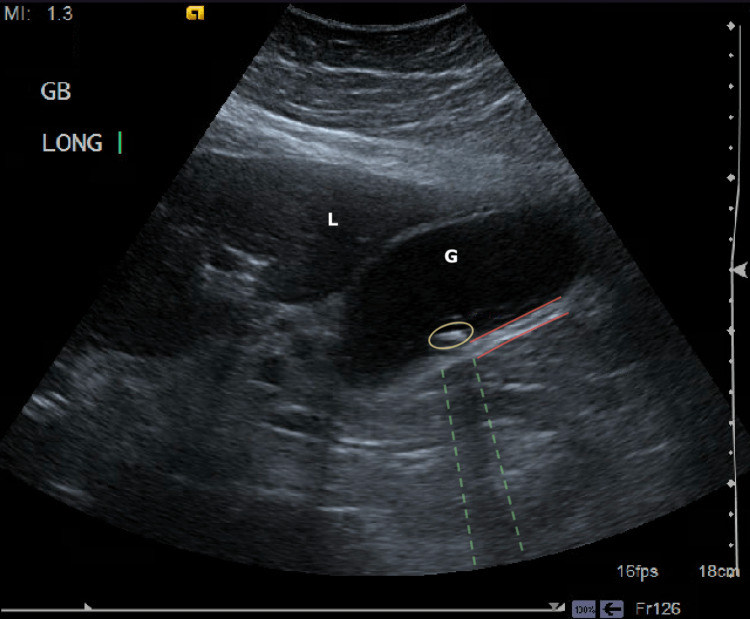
Ultrasound of the gallbladder longitudinal plane with the findings of cholelithiasis and acute cholecystitis Hyperechoic calculi are marked in the yellow circle, with acoustic shadowing seen in the dashed green lines. The red lines outline the thickened gallbladder wall at 4.8 millimeters (mm) (G: gallbladder; L: liver).
